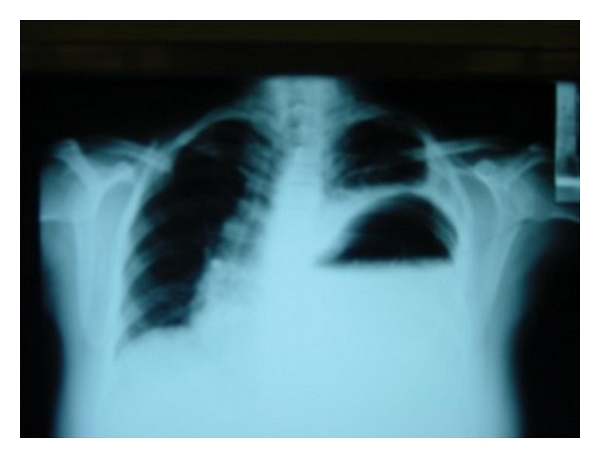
Chest radiograph showing the stomach in the left thoracic cavity.
